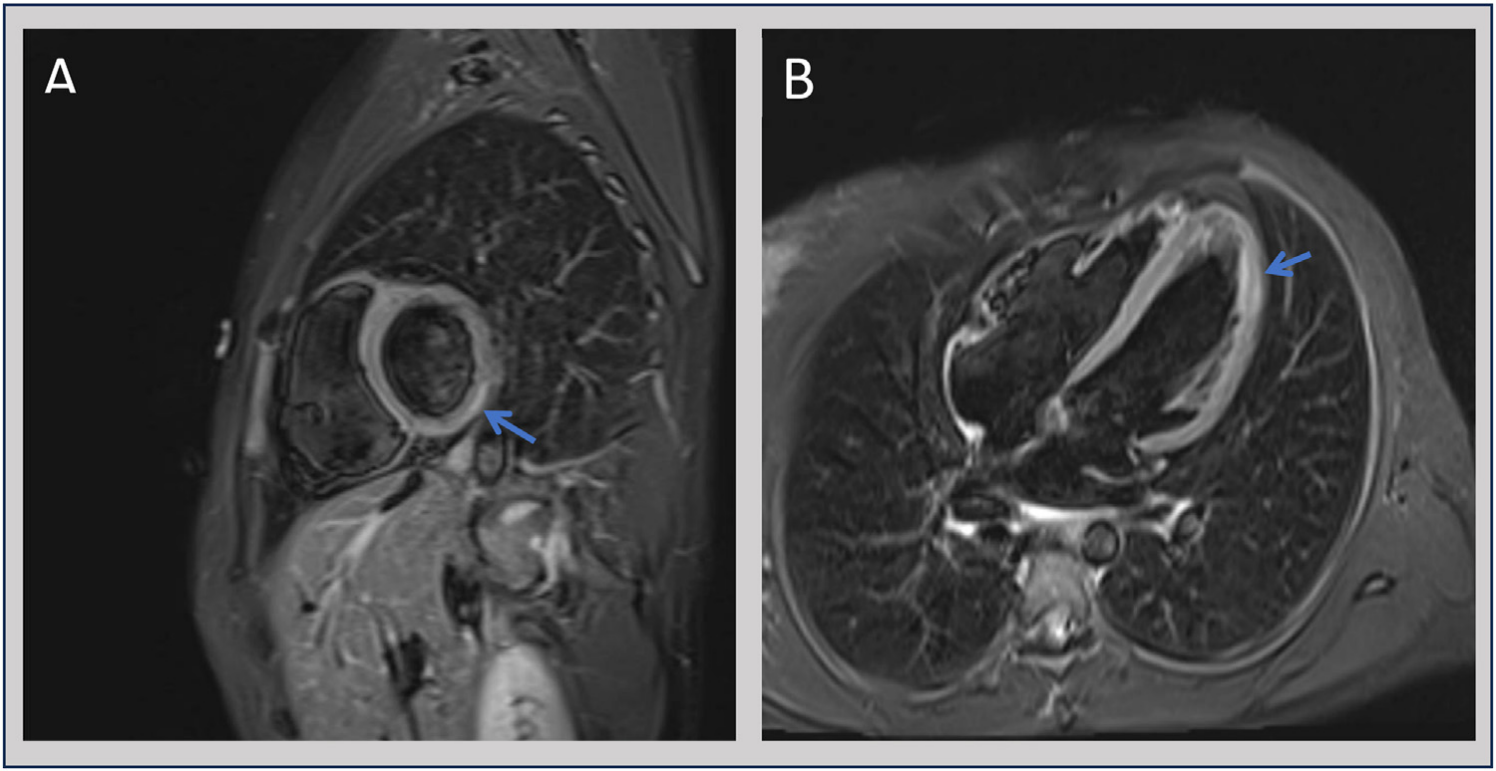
Cardiac magnetic resonance imaging (CMR) showing mild subepicardial oedema in the basal inferolateral myocardial wall (blue arrows) and corresponding late gadolinium enhancement (LGE) at the same segment, with no evidence of ventricular dilatation or fibrosis. Image A: short axis view. Image B: four-chamber view.
